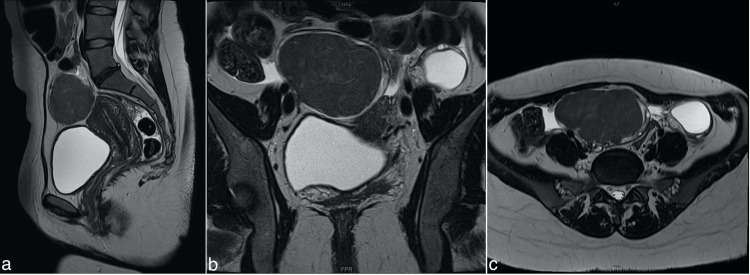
MRI pelvis (1.5 T Magnetom Sola, Siemens Healthineers®) Sagittal (a), coronal (b), and axial (c) TSE T2 images in high resolution show a soft tissue mass anterior, cranial, and just to the right of the uterus with surrounding free abdominal fluid. MRI: magnetic resonance imaging
